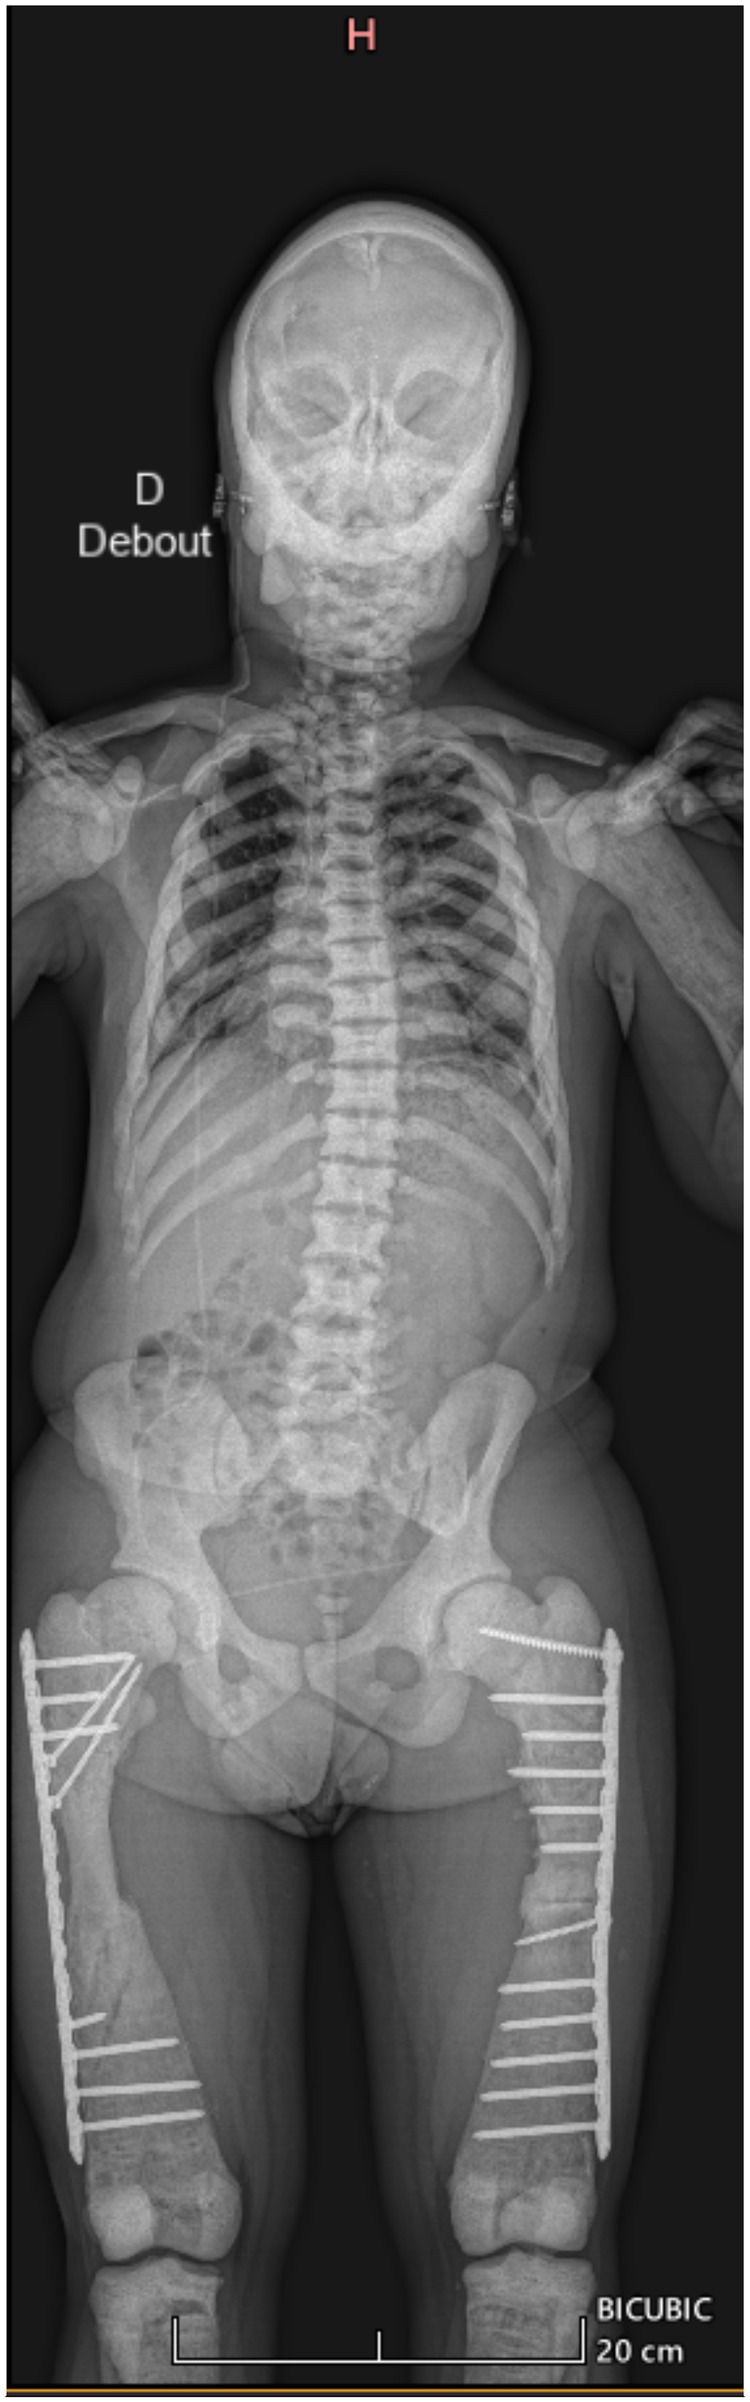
AP projection radiograph of a 22-year-old patient, 3 months after a right femur osteotomy with axis correction and right femur osteosynthesis (plate and screws).the patient's right lower limb reveals the previous year's procedure: the open reduction of an intertrochanteric fracture, fixed with double screws, and percutaneous minimally invasive osteosynthesis of the right femur (curved femoral plate with 13 holes, 247 mm).
